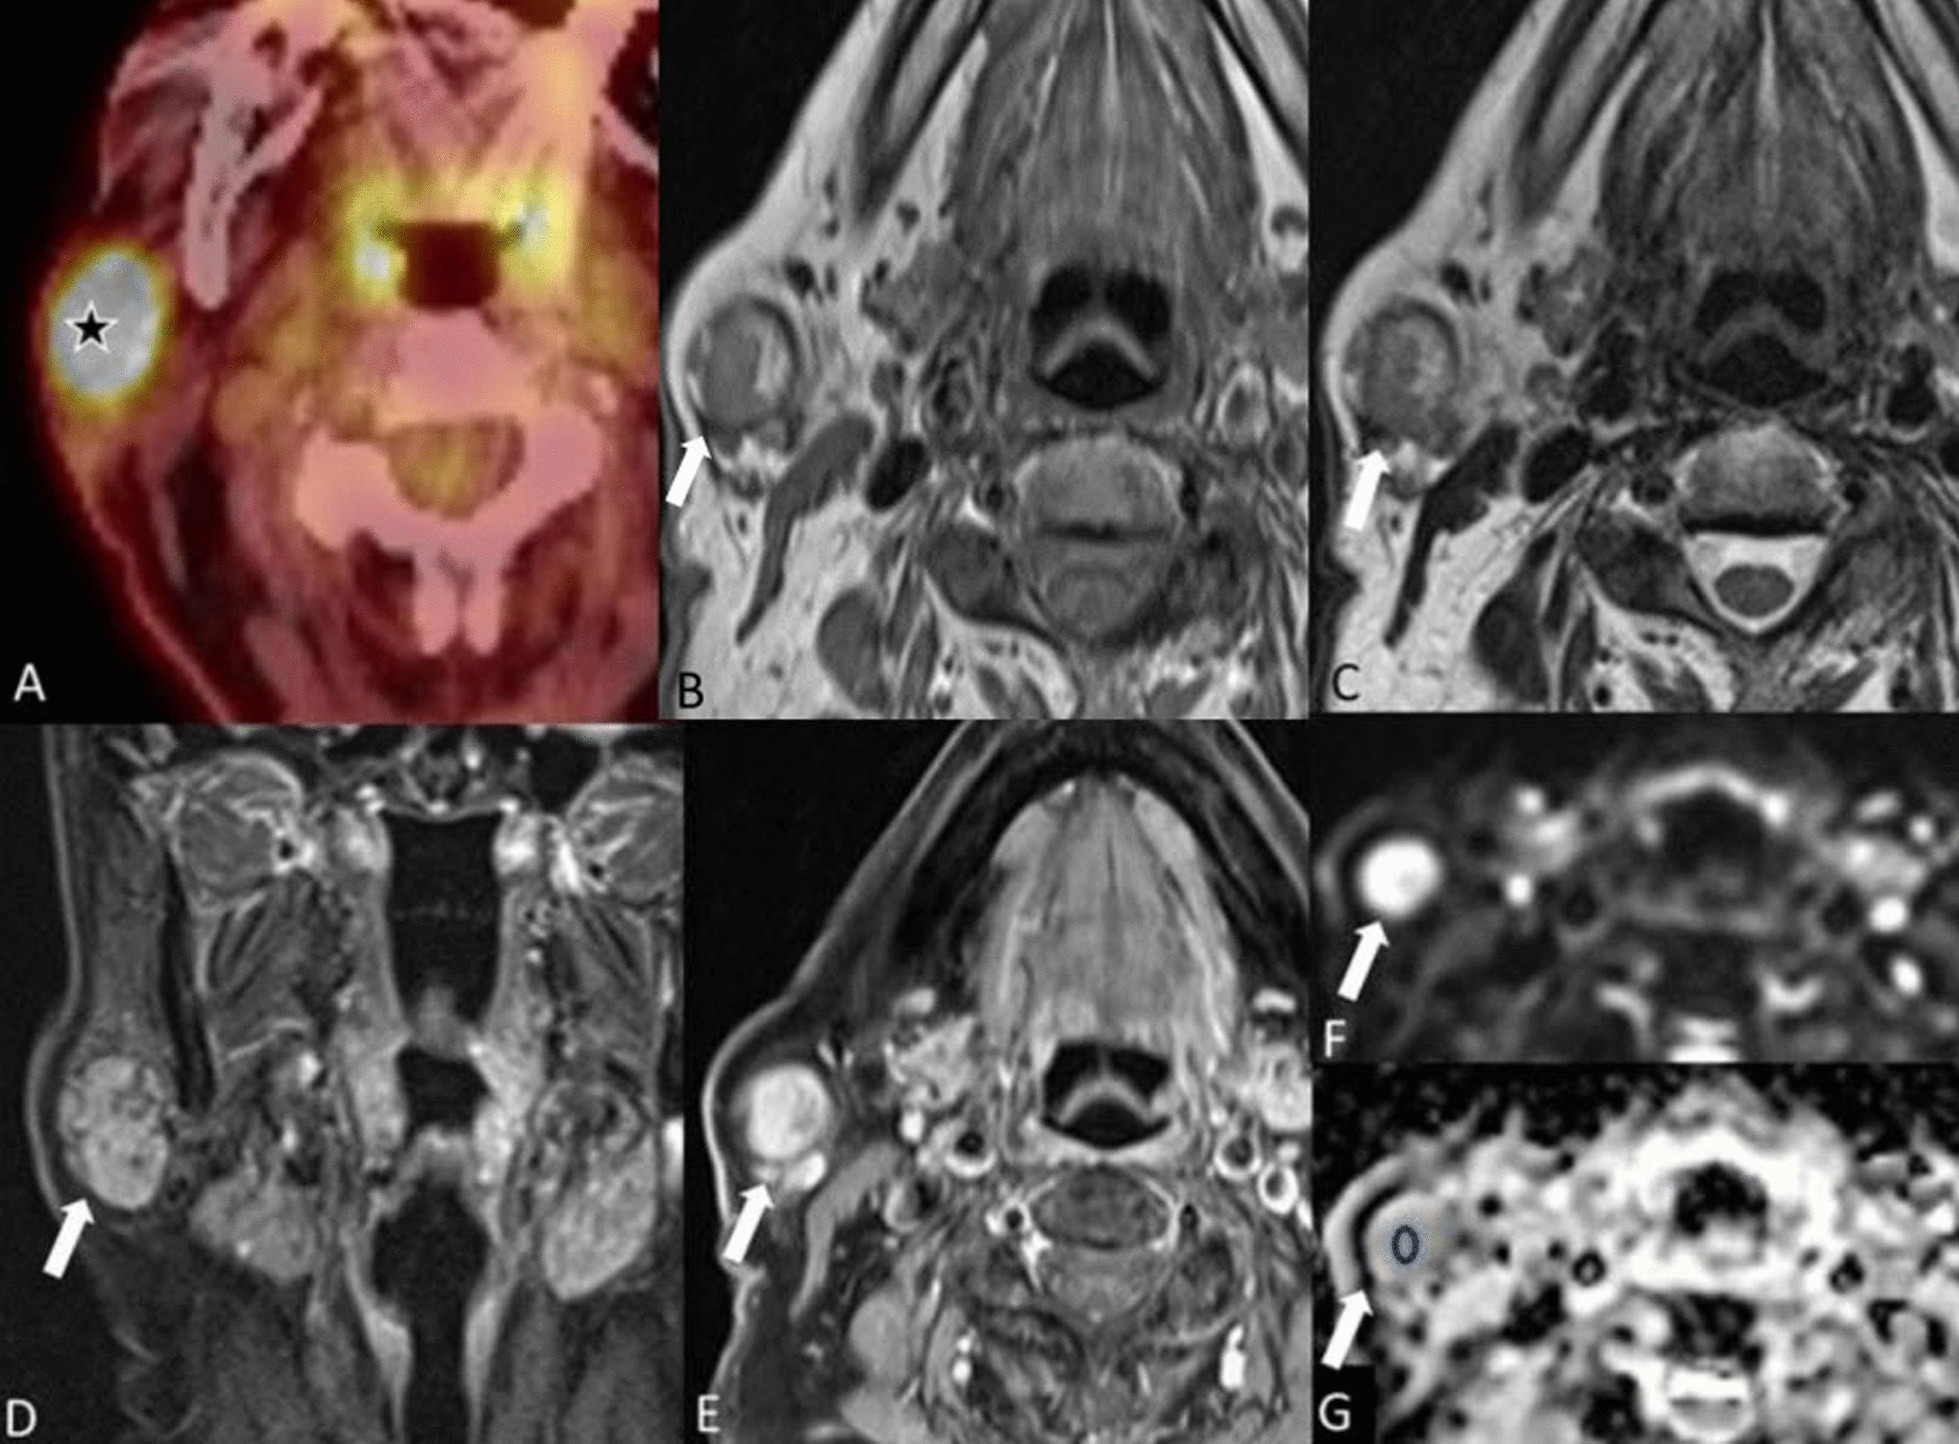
Oncocytoma. An 83-year-old female with melanoma of the right arm. ^18^F-FDG PET/CT (A) revealed a strongly glucose avid lesion in the right parotid gland. The lesion shows heterogeneous signal intensity on both T1- (B) and T2-w (C) images. The coronal turbo inversion recovery magnitude (TIRM) image (D) shows the slightly lobulated structure. There is heterogeneous enhancement of the lesion on the T1-w fat saturated image after gadolinium injection (E) and slight diffusion restriction as seen on the b800 image (F) with high signal intensity and corresponding low signal intensity (o) in the ADC map (G)
